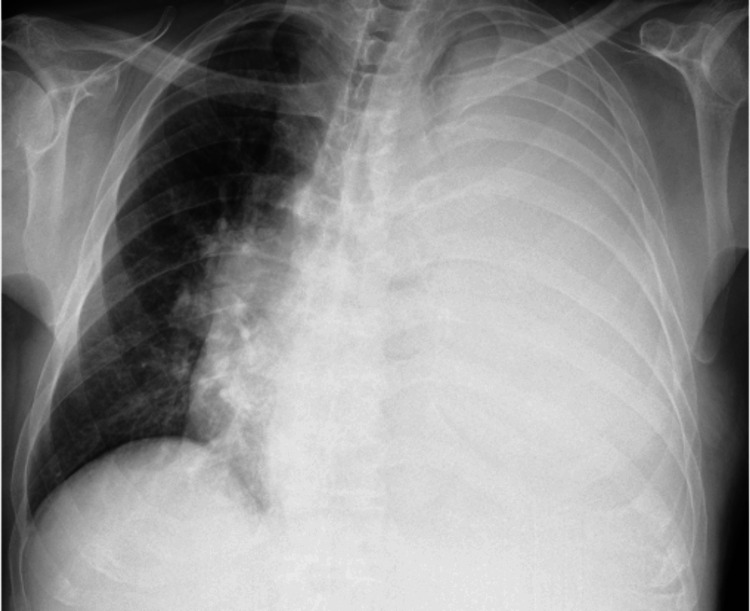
Chest radiograph (orthostatic position) showing a pleural effusion in the left lung field.
